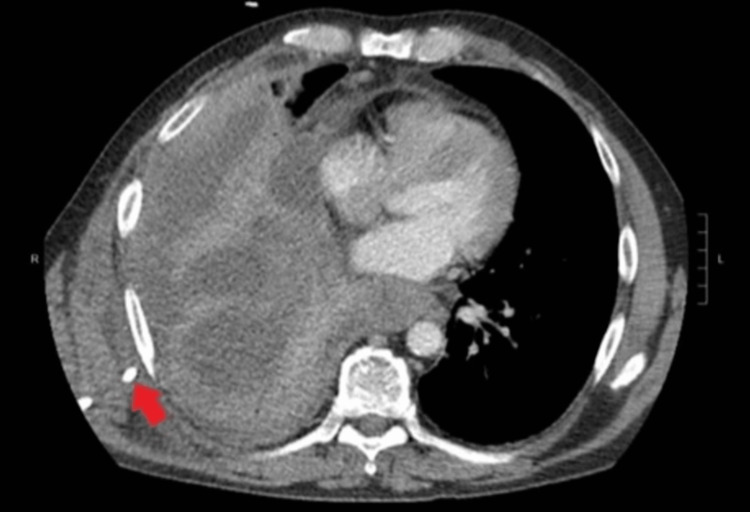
Chest CT with intravenous contrast on the fourth day of admission. Large multiloculated right-sided pleural effusion with associated atelectasis and pleural catheter (red arrow).
